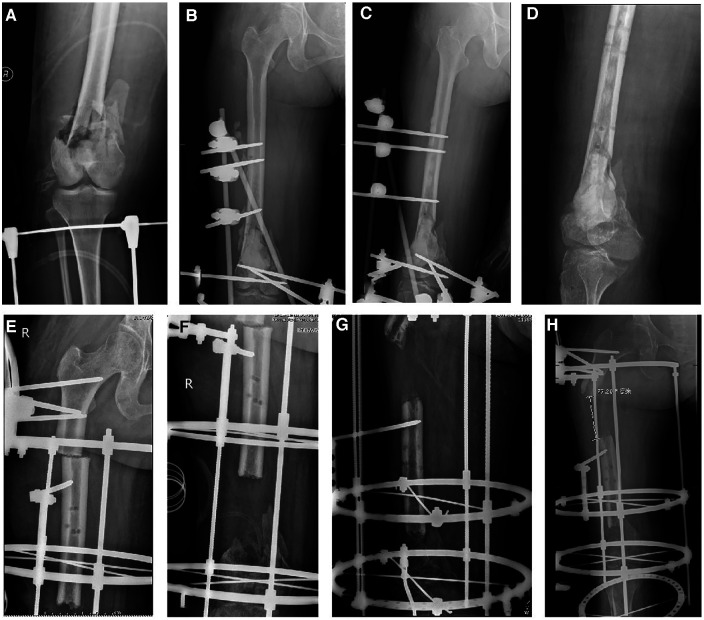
A 52-year-old female suffered from a serious open fracture and caused large segmental femoral defects. (A) x-ray images at initial injury; (B,C) x-ray images after debridement and filling with bone cement; (D) x-ray images after removal of the temporary external fixator; (E,F) x-ray images when bone transport frame was installed; (G,H) Poor osteogenesis occurred after 15 months of bone transport.
